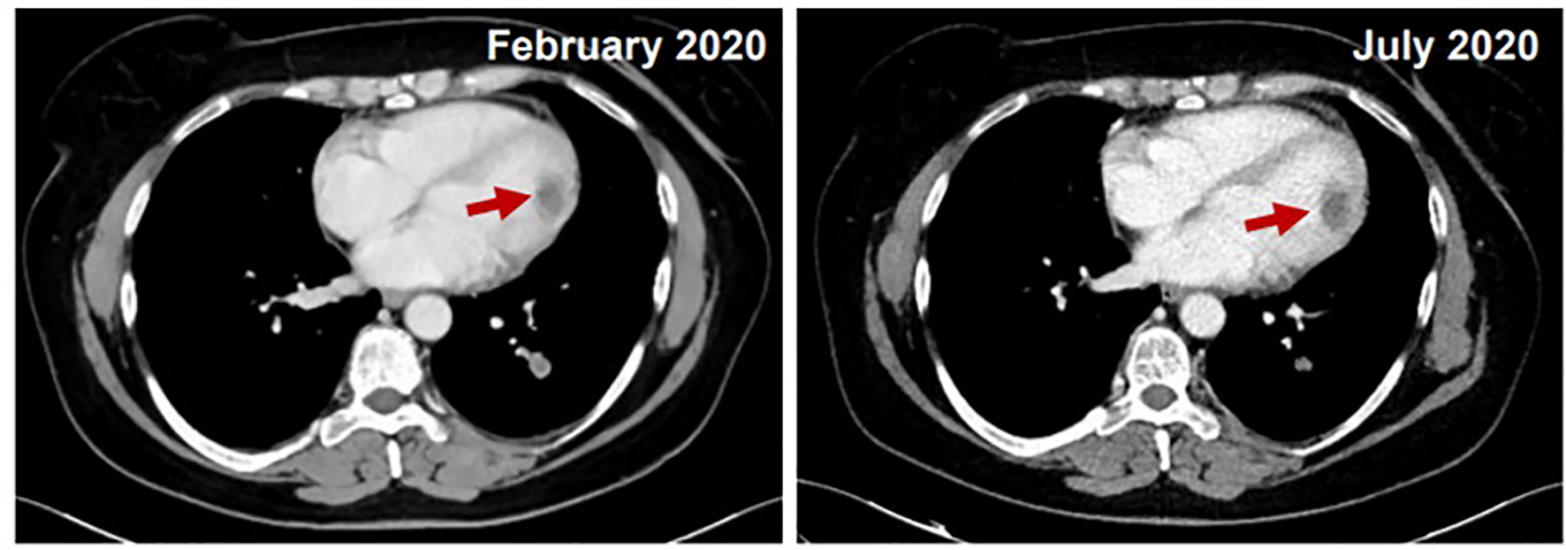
Comparison between February 2020 (on the left) and July 2020 (on the right): CT stability of cardiac mass and pulmonary and renal partial response.
